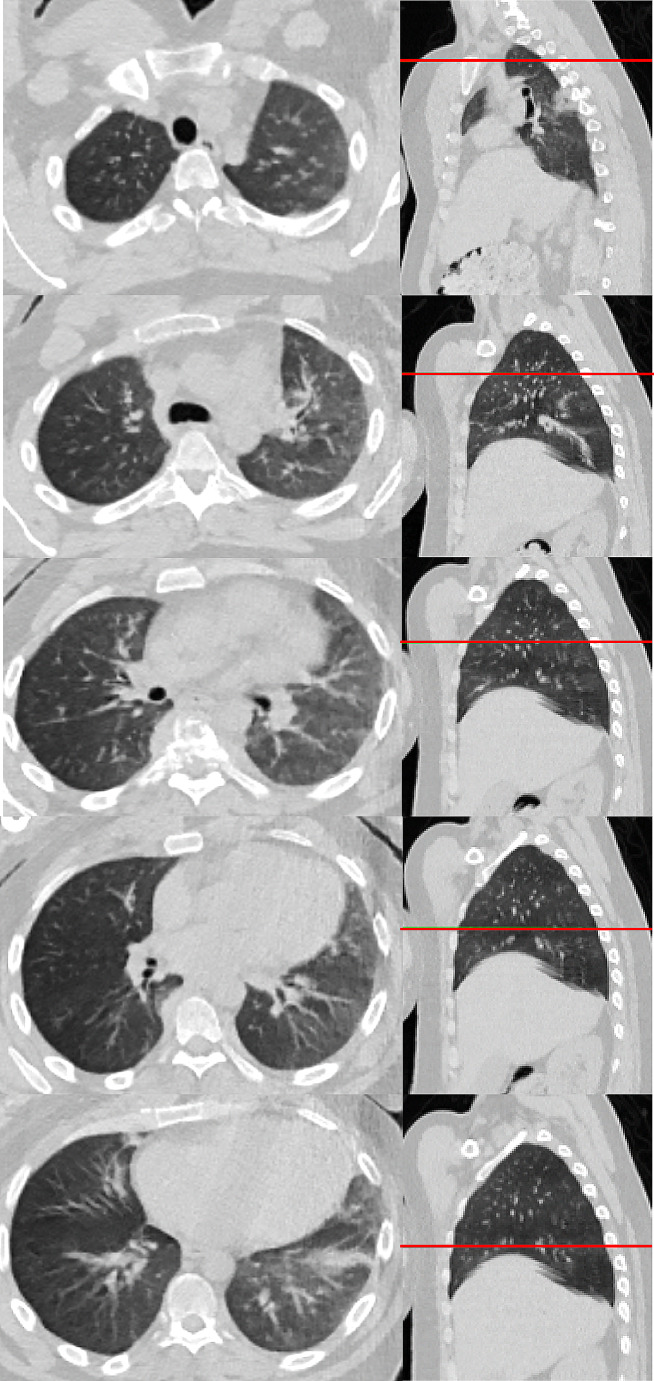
CT scan of the chest (lung-window) at different levels from cranial to caudal. Left side: axial view. Right side: saggital view with corresponding marking of level (red line)
